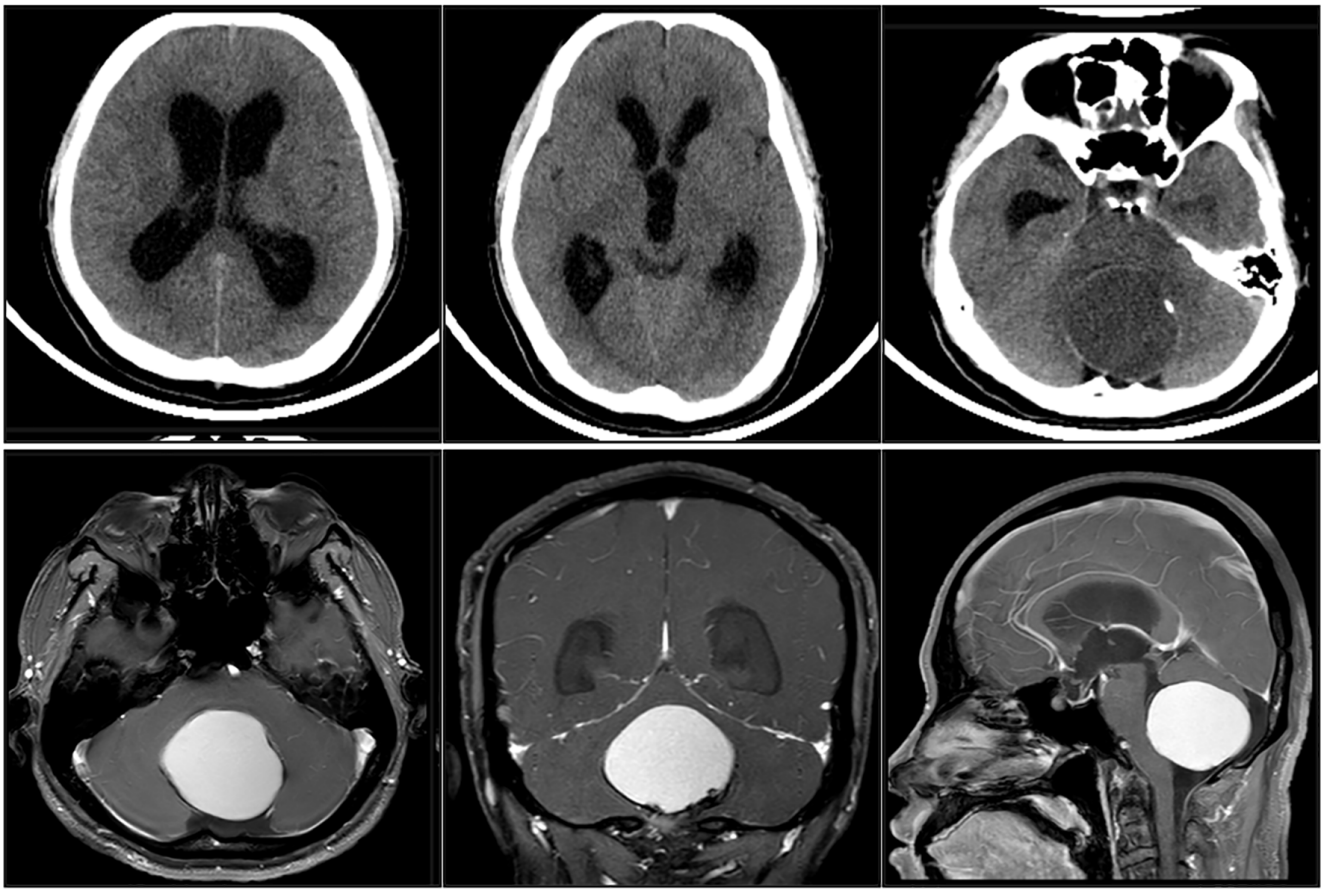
Admission cranial CT examination.
